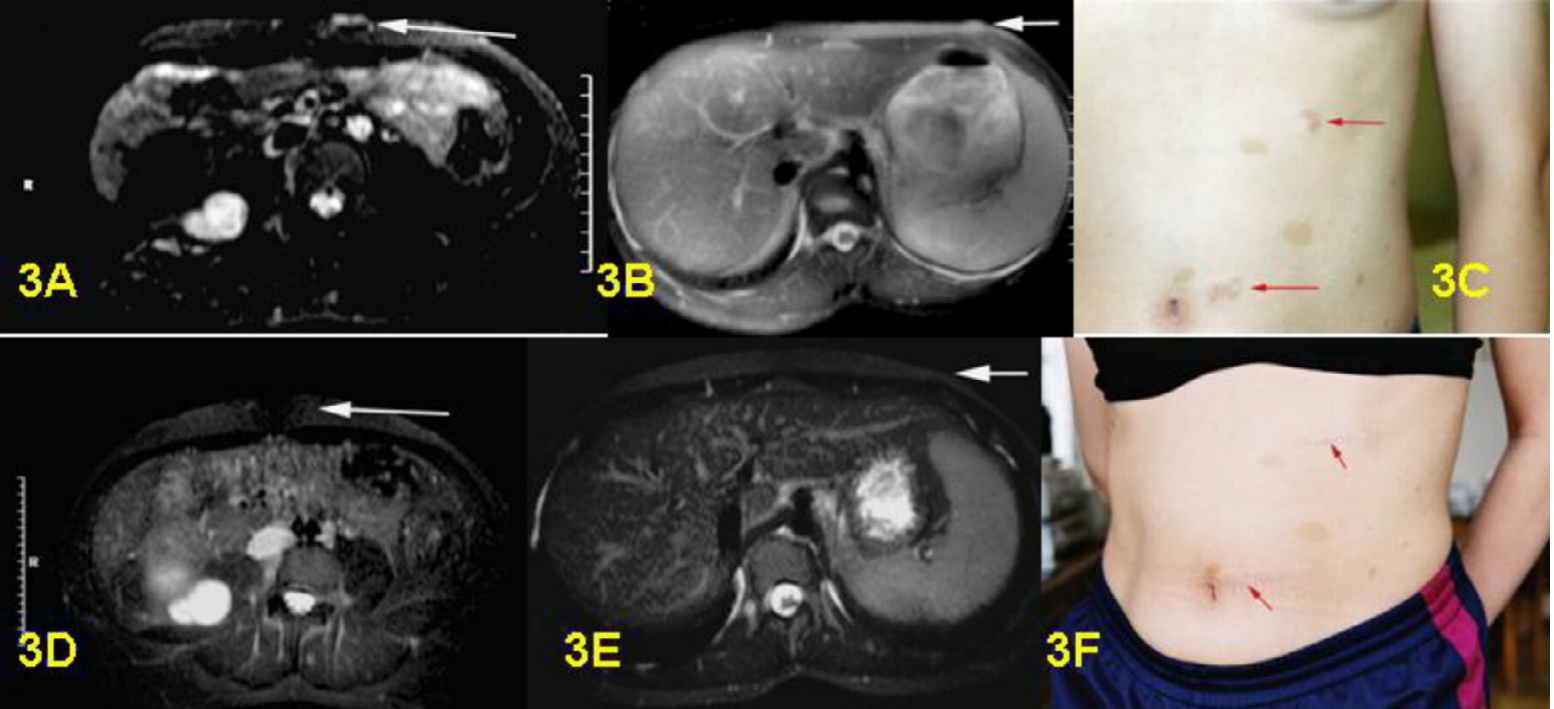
3A: Pre-surgical T2-weighted STIR sequence of left ventral abdominal wall, paraumbilical. Bright plexiform neurofibroma with thickening of cutis without involvement of muscle. 3B: Pre-surgical T2-weighted STIR sequence, axial section of left ventral abdominal wall, costal margin. Small bright plexiform neurofibroma in cutis and subcutis ventral left. 3C: Pre-surgical clinical frontal view of abdomen with both superficial plexiform neurofibromas visible. 3D: Post-surgical MRI control from A. Complete removal of plexiform neurofibroma. 3E: Post-surgical MRI control from B. Complete removal of plexiform neurofibroma. 3F: Post-surgical clinical frontal view of abdomen. Only two visible scars left after surgery.
